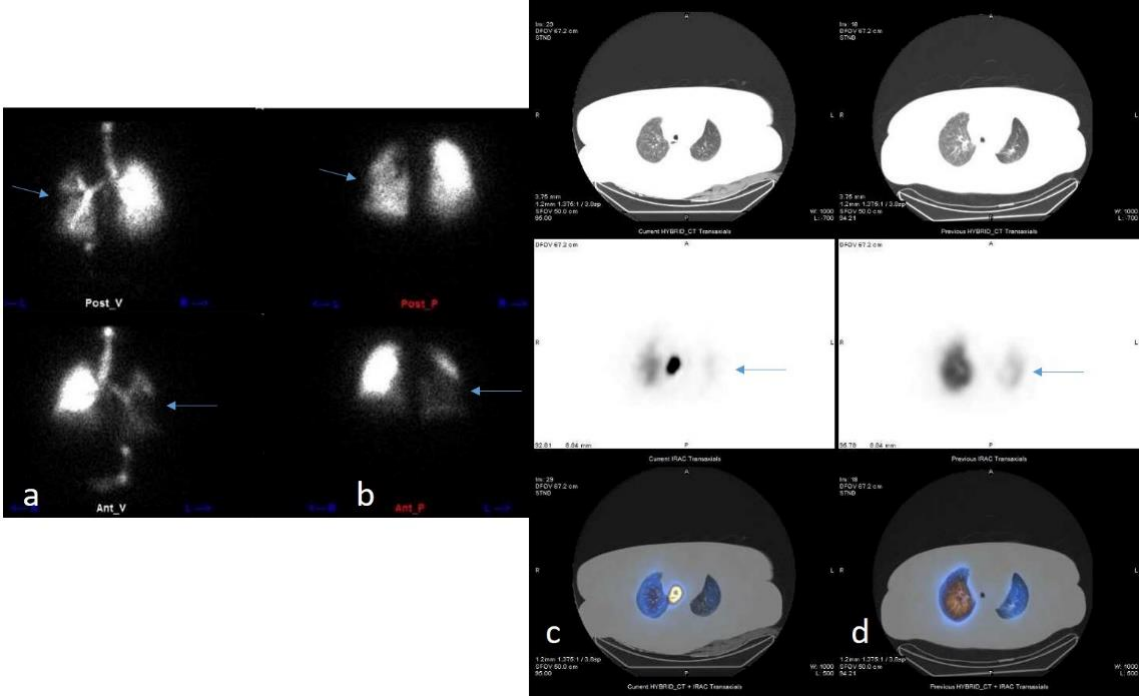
Lung planar V/Q images (a and b) and SPECT/CT V/Q images (c and d) of the patient. Matched perfusion/ventilation defects are observed in the left lung without any corresponding CT abnormalities, suggesting that the scan is not compatible with pulmonary embolism (PE). The blue arrows indicate the regions where these matched defects are visible, highlighting areas of concern that correspond to both perfusion and ventilation defects
